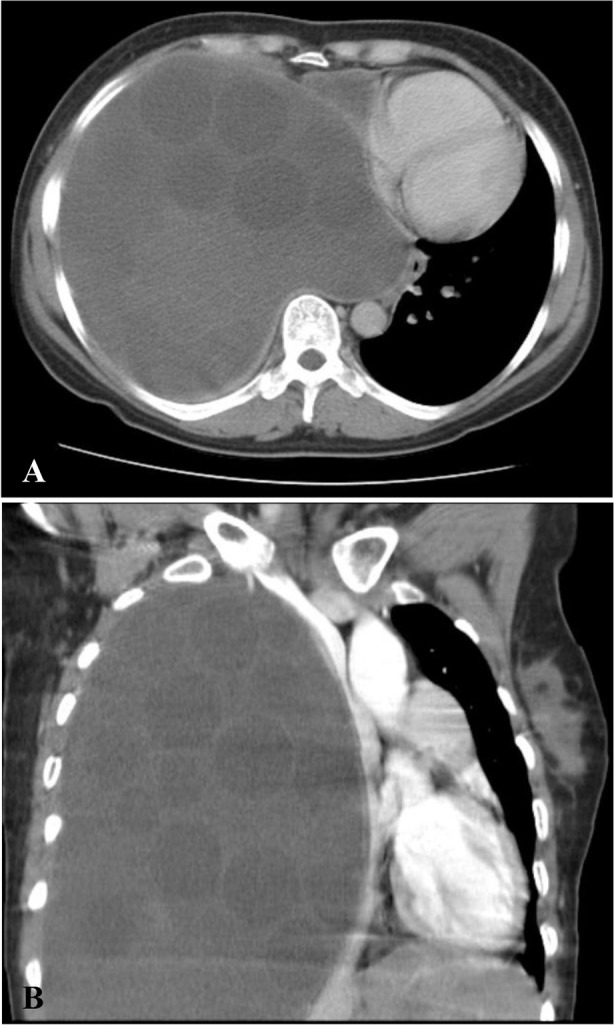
(A) Computed tomography showed multiple cysts filling the entire right pleural area and mediastinal shifting to left. (B) Coronal view computed tomography showed the same finding.
